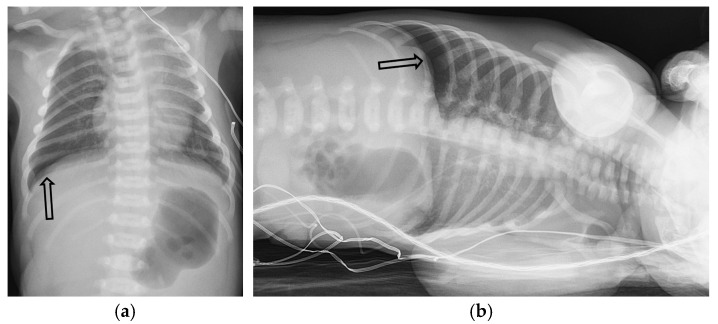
One-day-old term neonate presents with tachypnea. Frontal chest radiograph (a) demonstrates a small right pneumothorax (open black arrow). Left lateral decubitus frontal chest radiograph (b) reveals increased conspicuity of small-to-moderate right pneumothorax (open black arrow).
